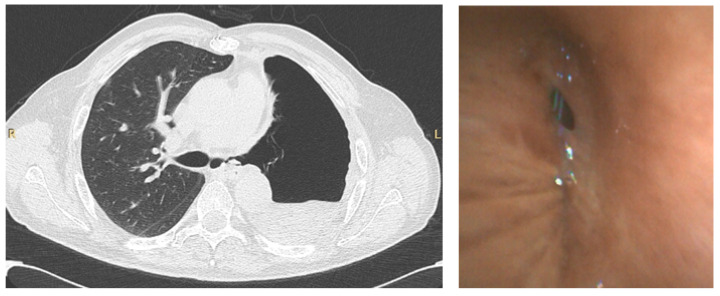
CT scan (left) of a patient that underwent left pneumonectomy, showing a possible left bronchopleural fistula, in a COVID-19 positive patient, confirmed by bronchoscopy (right) using single-use flexible bronchoscope.
